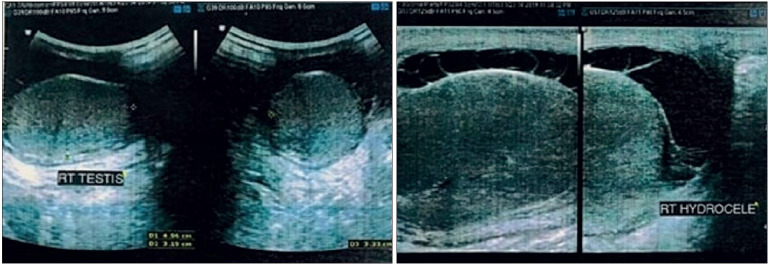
Ultrasound of scrotum shows both testes normal in size, shape and echotexture. Right scrotal sac shows mild free fluid with multiple fine internal septations.
